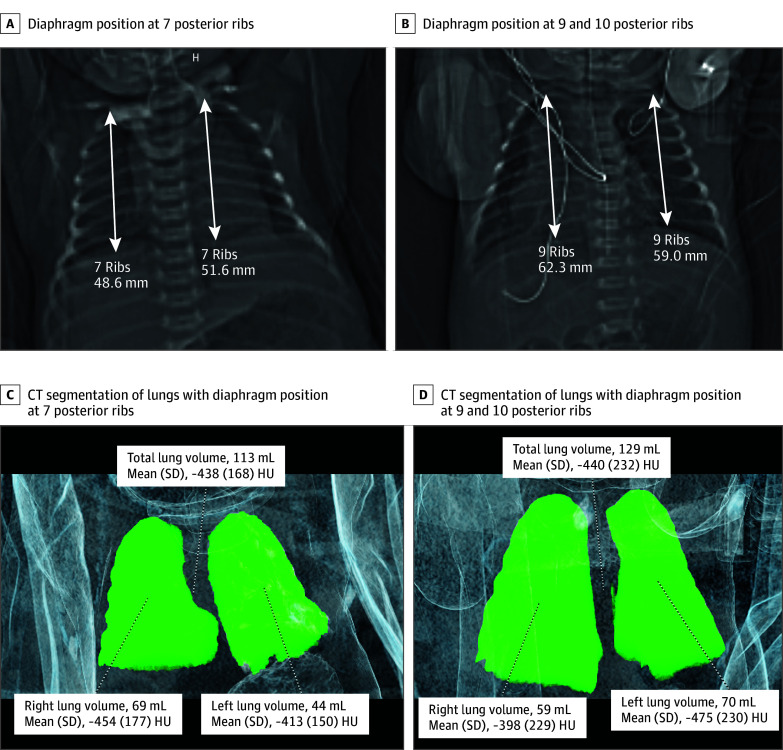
Chest Radiograph Equivalent for an Infant Chest radiograph equivalent for an infant with diaphragm position at 7 posterior ribs (A) and 9 and 10 posterior ribs (B) to summarize the association of diaphragm position with posterior rib. The diaphragm position was defined as the last posterior rib that completely intersects the diaphragm or had the diaphragm positioned entirely within the posterior rib. The rib above was used if no posterior rib intersected the diaphragm. When the diaphragm involved 2 posterior ribs but did not completely transect the lower rib, the upper rib was used if less than 50% of the intercostal space between ribs included lung fields and vice versa as seen in panel B (right chest) (eMethods and eFigure 1 in Supplement 1). Apex-diaphragm distance also shown. Total, left, and right lung volume and Hounsfield units (HU, a quantitative measurement of radiodensity) measurements from computed tomography segmentation of the lungs in each respective infant (C and D). Despite the different diaphragm positions, the absolute lung volume were similar.
